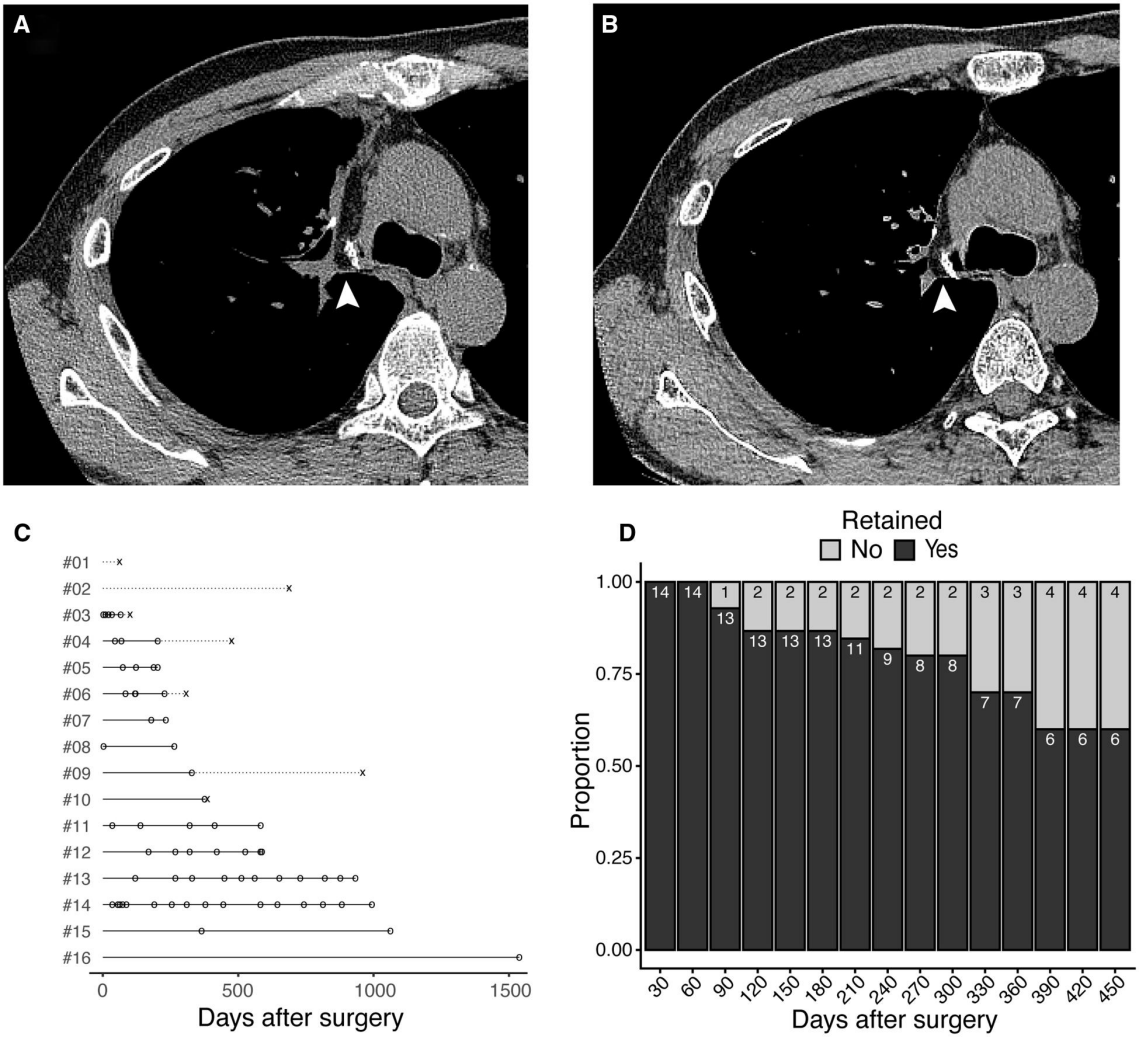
Retention rate of the pedicled thymic flaps after surgery. (A, B) Postoperative CT scans showing the retention of the flap adjacent to the bronchial stump (arrowheads) at postoperative days 35 (A) and 413 (B). (C) Swimmer plot showing days of the CT scan. A circle (o) represents the day of the CT scan with a retained flap, and a cross (×) represents the day of the earliest CT scan with a regressed flap. Solid lines represent the periods with a high probability of having a retained flap, and dotted lines represent the period during which the flap has possibly regressed. (D) Bar plot showing the proportion of the retained and regressed flaps at each time point after surgery
